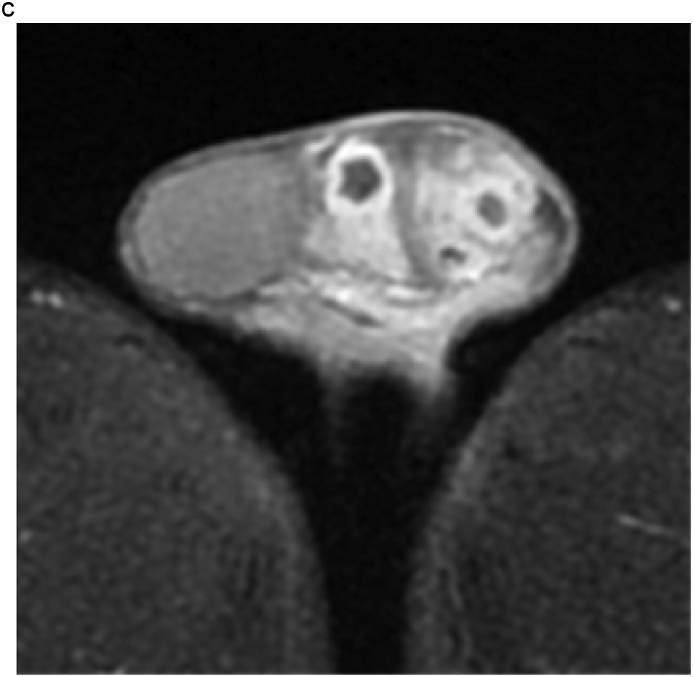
(a) Multiple hypoechoic regions and marginal Doppler signal enhancement are visible. (b) T2-weighted MRI showing multiple nodules with low signal intensity versus the normal testis. (c) In the early enhancement phase of T1-weighted MRI, marginal enhancement is prominent.
